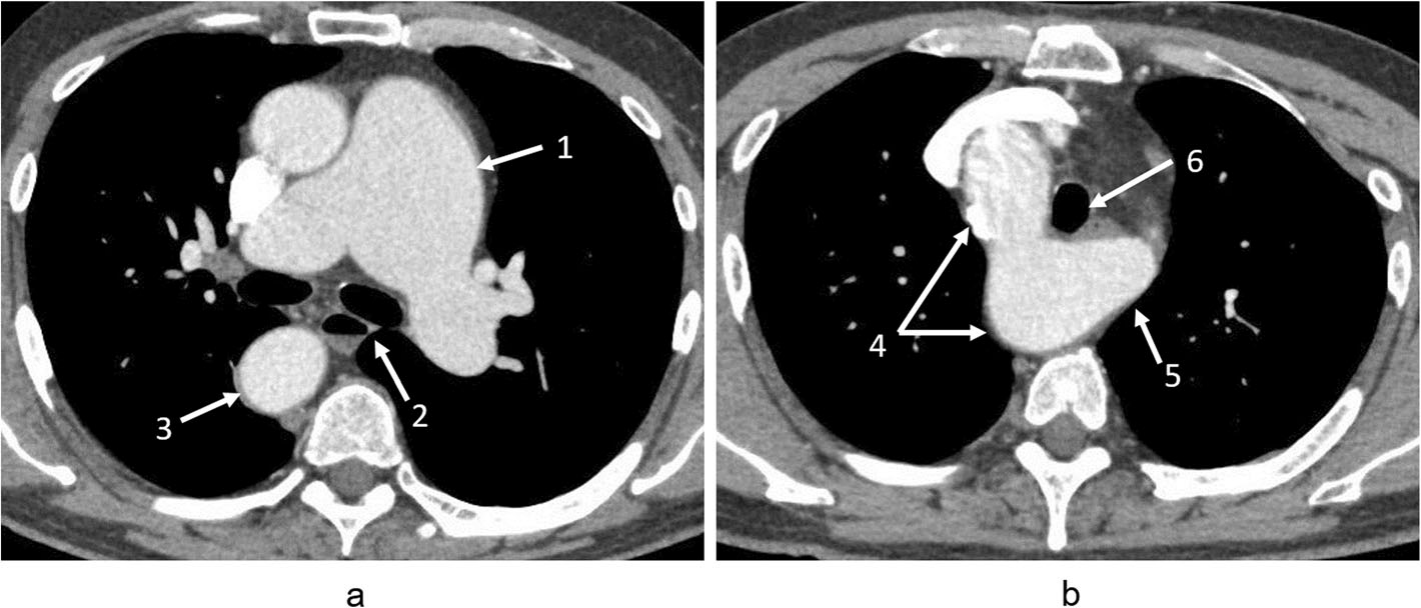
a Contrast computed tomography showing a dilated pulmonary trunk. The dilated part of the pulmonary trunk (arrow 1) is not in contact with the sternum, nor does it displace the mediastinal tissues (arrow 2). The descending aorta is depicted in the right posterior mediastinum (arrow 3). b Arrow 4 indicates the right-sided aortic arch. The Kommerell’s diverticulum (3 cm) is depicted at the origin of the left subclavian artery (arrow 5). The trachea is slightly displaced by the diverticulum (arrow 6)
